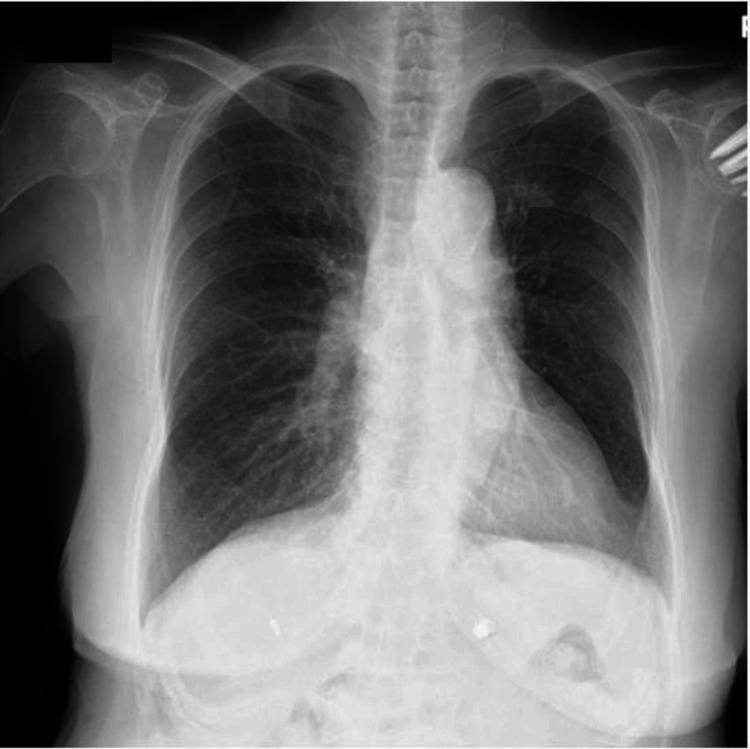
Chest radiograph in case 2. Chest radiography showed a cardiothoracic ratio of 52%.
